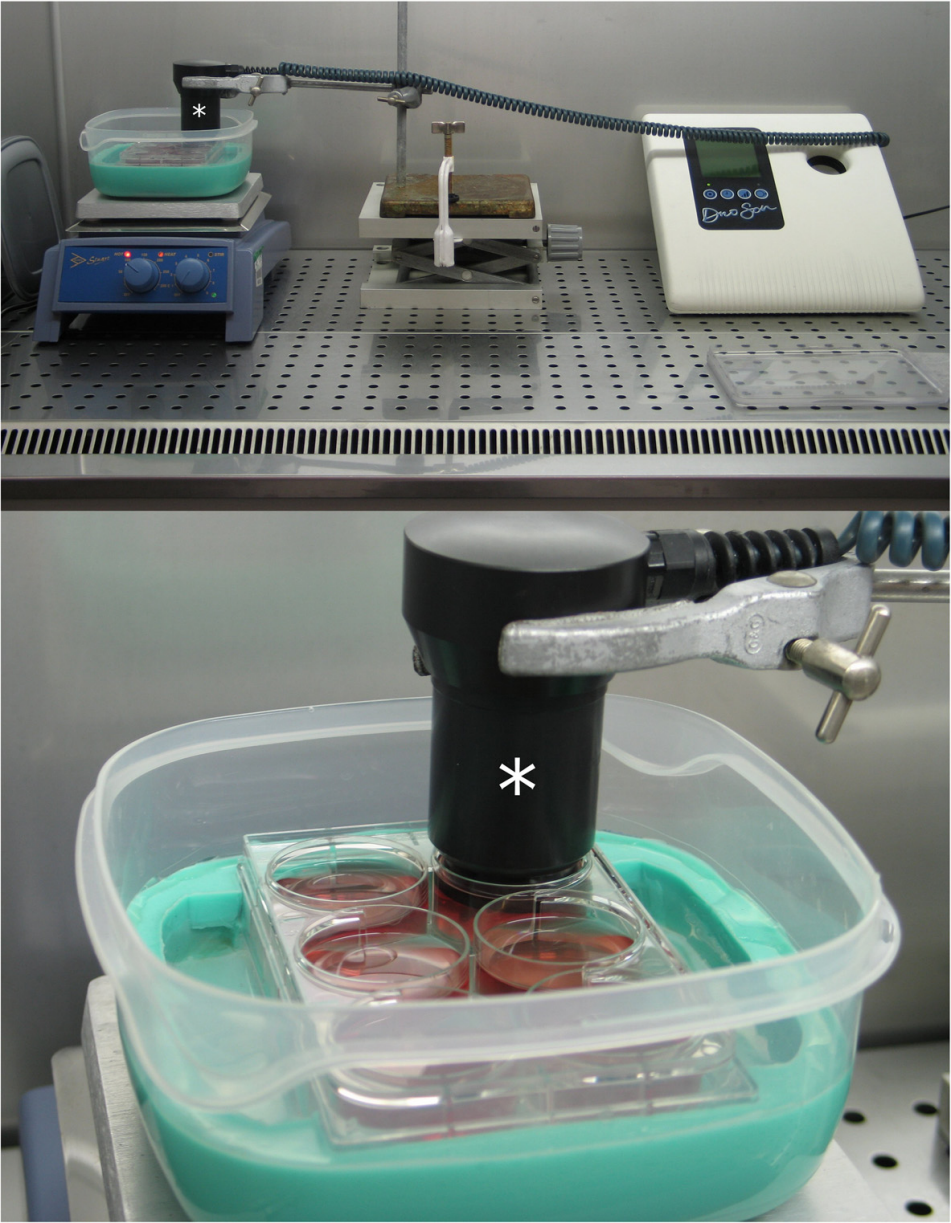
Treating biological cells with ultrasound in multiwell culture plates. DuoSon with transducer (identified by *) clamped in position in a laminar flow hood (*top*) and a close-up of a six-well plate supported by silicone in a water bath with the transducer submerged in culture media (*bottom*)
